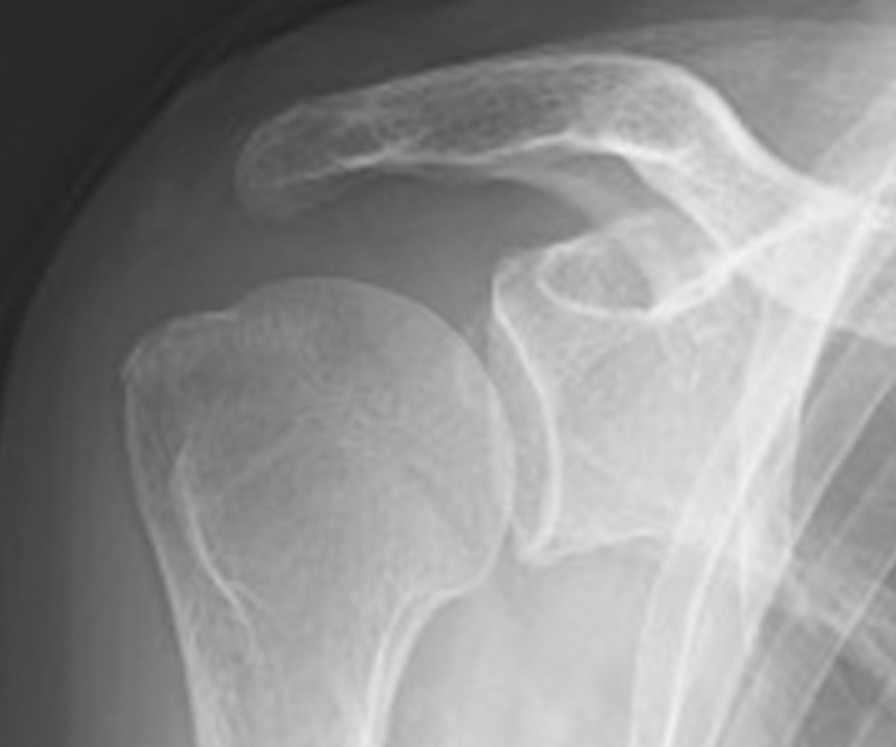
Anteroposterior radiograph of the right shoulder of a 71-year-old Asian woman. There is no apparent subacromial spur
